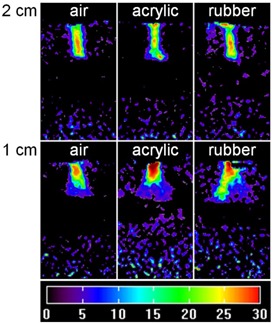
Temperature maps in *ex vivo* muscle tissue for sonications targeted at both 2 and 1 cm depth below the interface of air, acrylic, and rubber. Ultrasound beam propagation direction is from bottom to top. All temperatures (°C) displayed as relative change from baseline.
